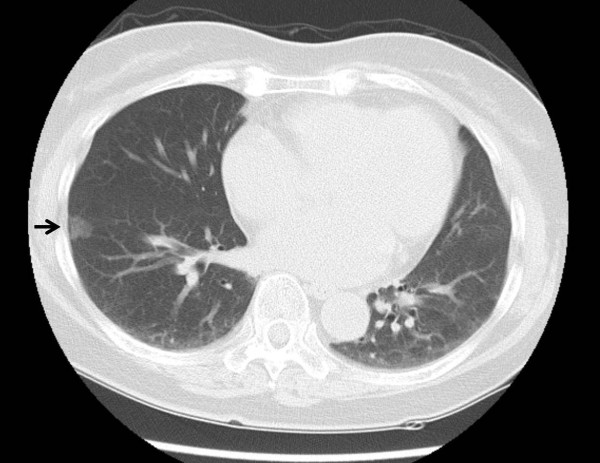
Chest CT revealed a tumor (arrow) in anterior basal segment of the right lower lobe (RS8).
